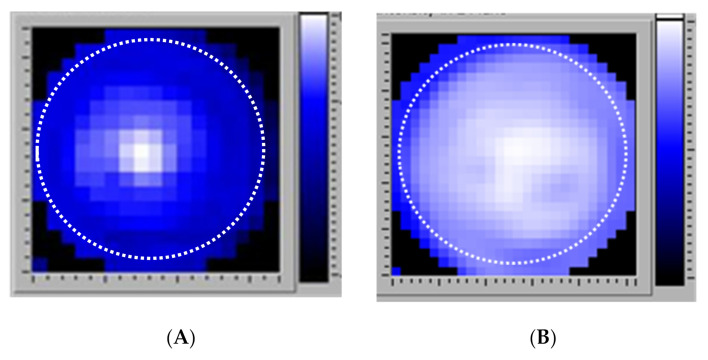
Ultrasound pressure distribution pattern before (A) and after (B) modifications. Dotted circles indicate the diameter of the hanging insert.
